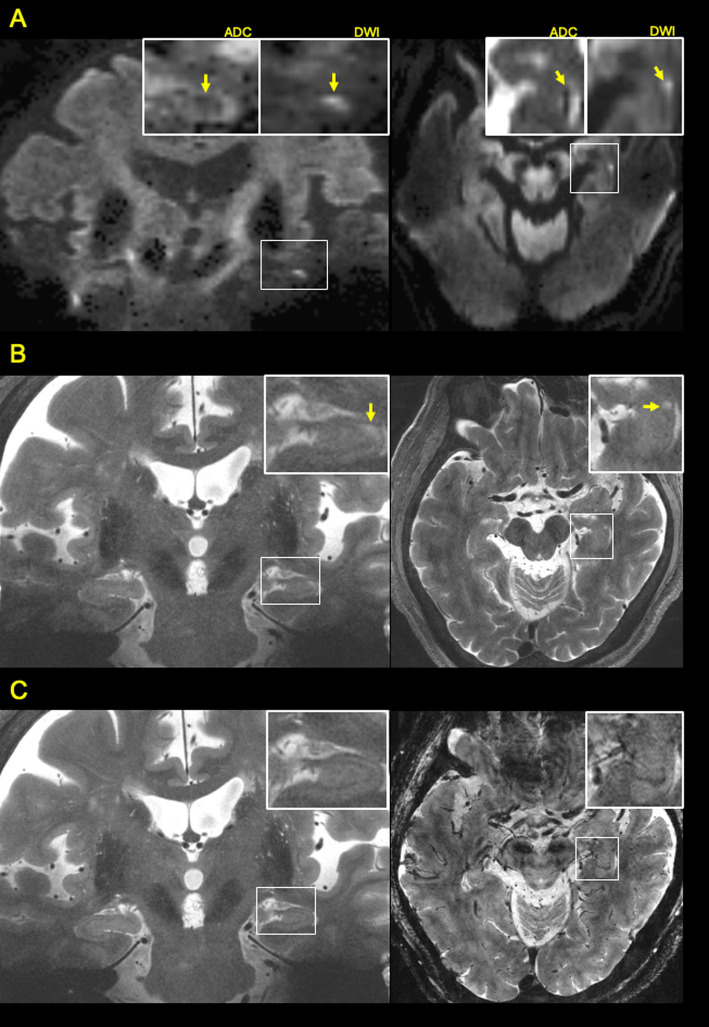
Serial 7 T MRI findings in Case 3. (A) Acute 7 T diffusion-weighted imaging (DWI) obtained 68 h after symptom onset demonstrates a punctate hyperintensity localized to the hippocampal CA1 region (arrow). Corresponding apparent diffusion coefficient (ADC) maps show focal hypointensity at the site of the DWI lesion, confirming restricted diffusion. Magnified views of the lesion are shown in the upper-right corner of each image; the left inset displays the ADC map, and the right inset displays the DWI image. (B) Corresponding 7 T T2-weighted imaging reveals a colocalized cortical-layer hyperintensity within CA1 (arrow), best appreciated on coronal reformats. (C) Follow-up 7 T T2-weighted imaging on day 18 demonstrates normalization of hippocampal signal with no residual hyperintensity.
